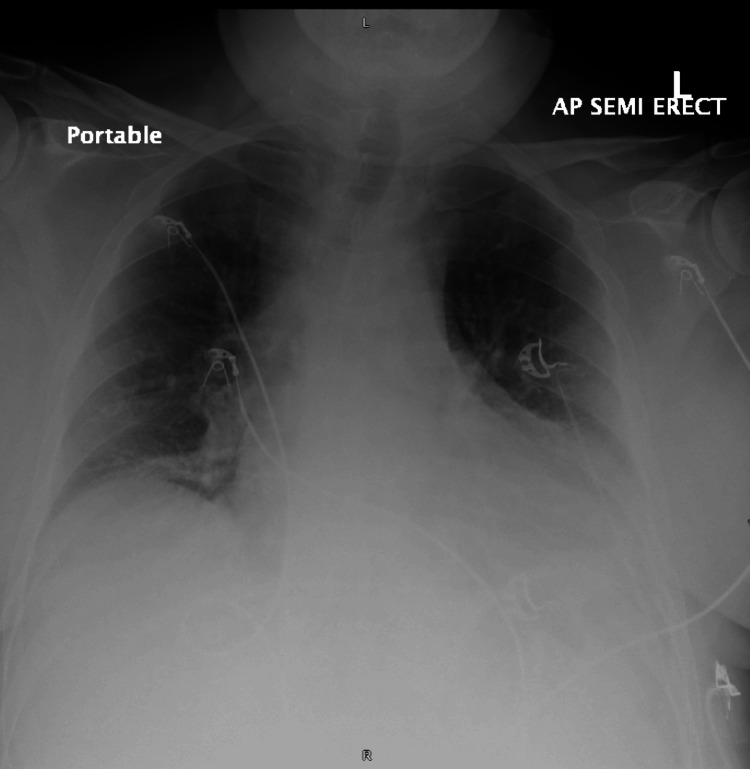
Portable chest X-ray in the resuscitation room
